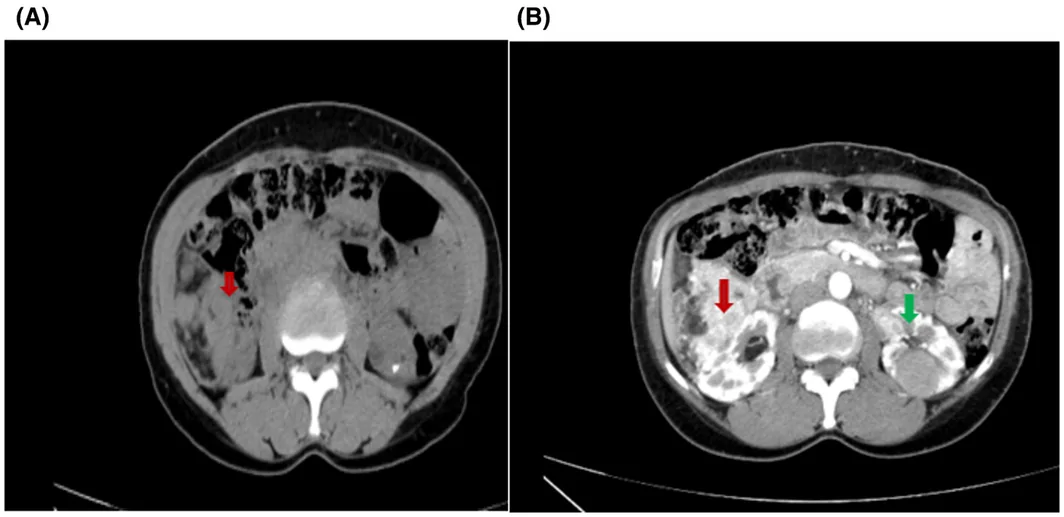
Imaging findings of the case of TSC* (A) Pre contrast Abdominal CT. (B) Post contrast CT of the abdomen showing bilateral renal cortical angiomyolipomas (AML), identified as hypo dense, enhancing right renal cortical mass with internal fat (red arrow). Additionally, there are two hyper dense, enhancing left renal cortical masses (green arrow). AML = angiomyolipoma; LAM = lymphangioleiomyomatosis.
